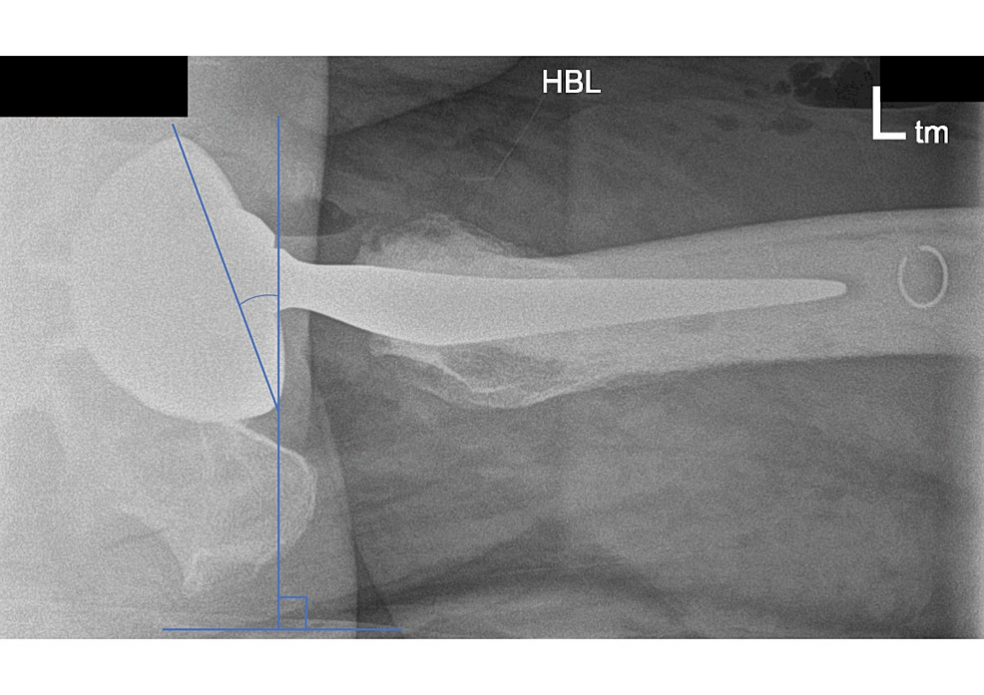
Radiographic cup anteversion angle measurement. The cup anteversion angle was calculated as the angle between a line touching the opening surface of the acetabular component and a line perpendicularly drawn to the table on cross-table lateral hip radiographs.
